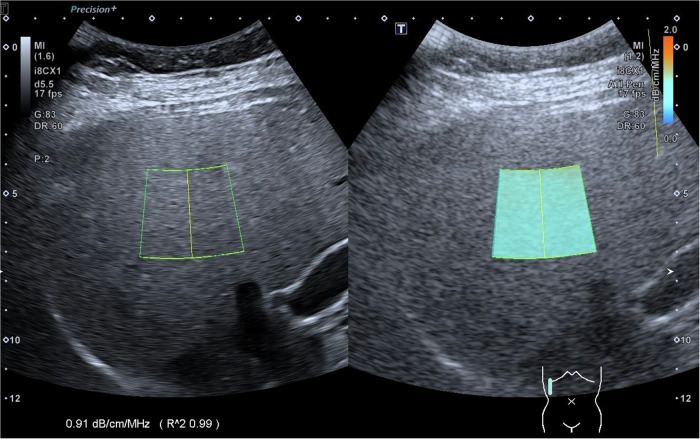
Performance of US ATI. A representative sonographic ATI image from a 10-year-old boy with hepatic steatosis, showing an ATI value of 0.91 dB/cm/MHz. The associated *R*² value of 0.99 indicates excellent reliability of the measurement
